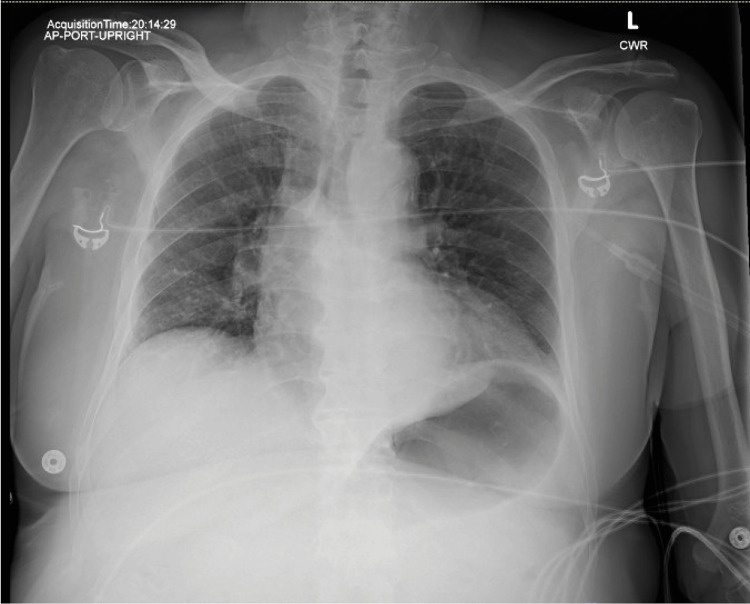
Anteroposterior chest x-ray on admission
